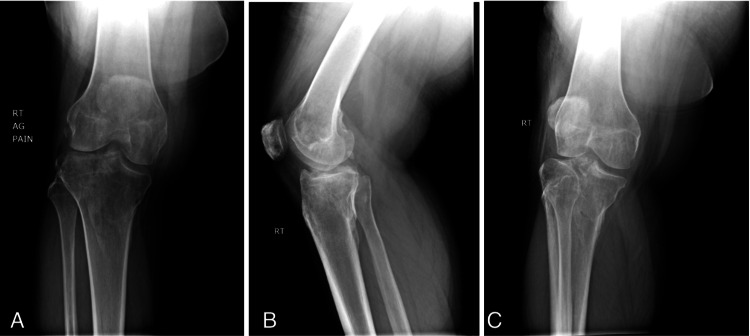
An anteroposterior (A), lateral (B), and oblique (C) X-ray of the right knee shows a complex bicondylar tibial plateau fracture with significant varus deformity after nonoperative management.
